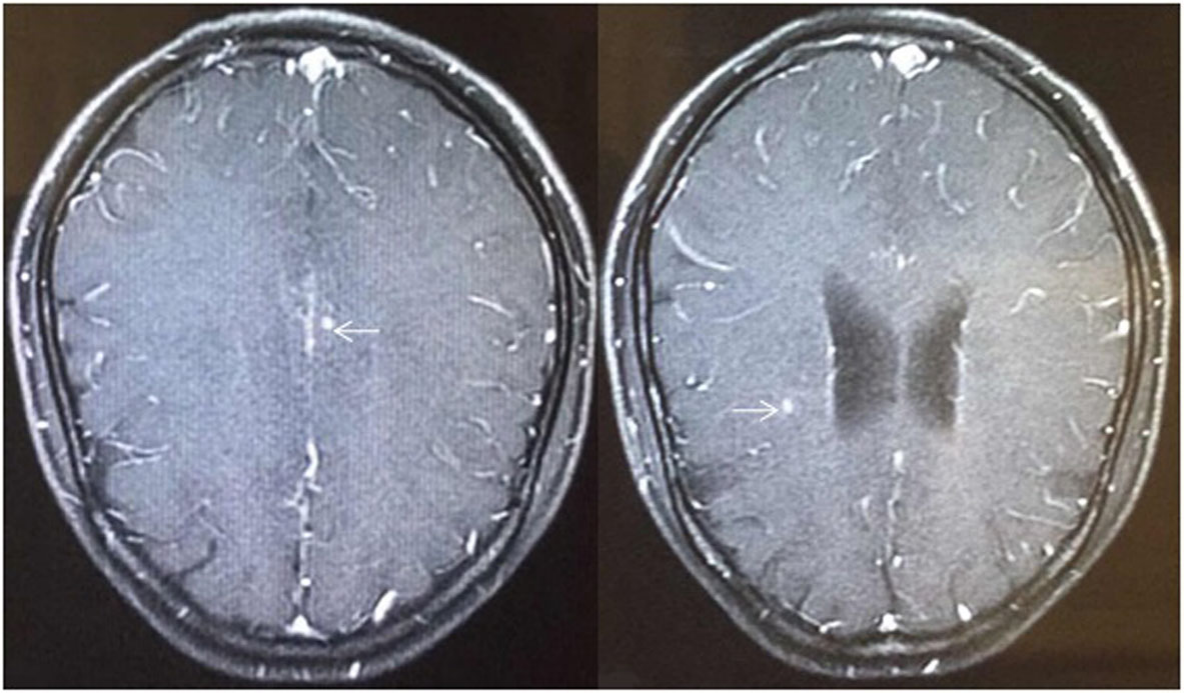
Cranial MRI showing bilateral centrum semiovale and multiple small plaques in the frontal lobe
